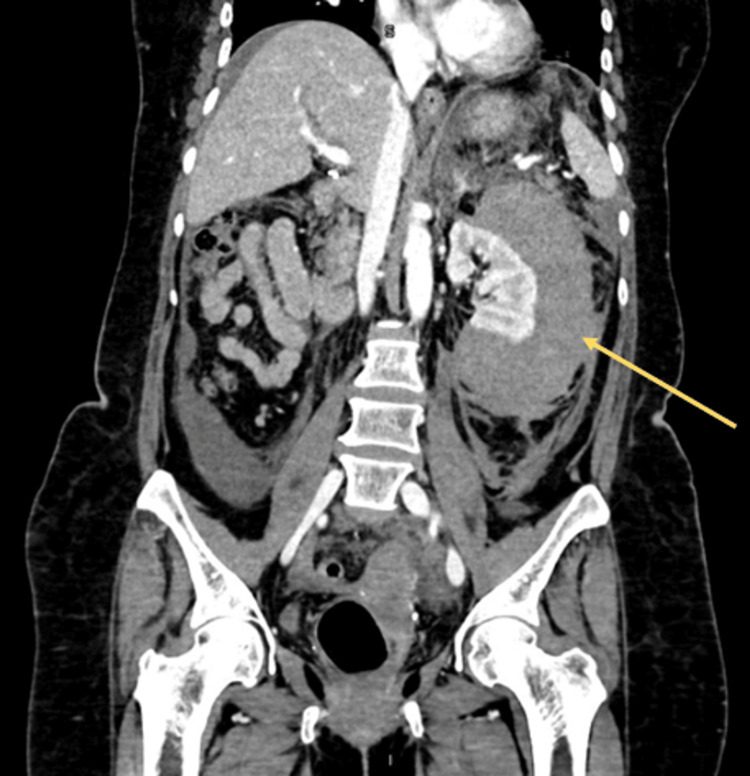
Contrast-enhanced abdominal CT (coronal view) Left kidney with a large hyperdense posterolateral collection (yellow arrow). Measurements approximately: 10 × 8.5 × 13.5 cm. Loss of left renal morphology. Obliteration of the perirenal fat stranding. No adjacent mass is observed.
